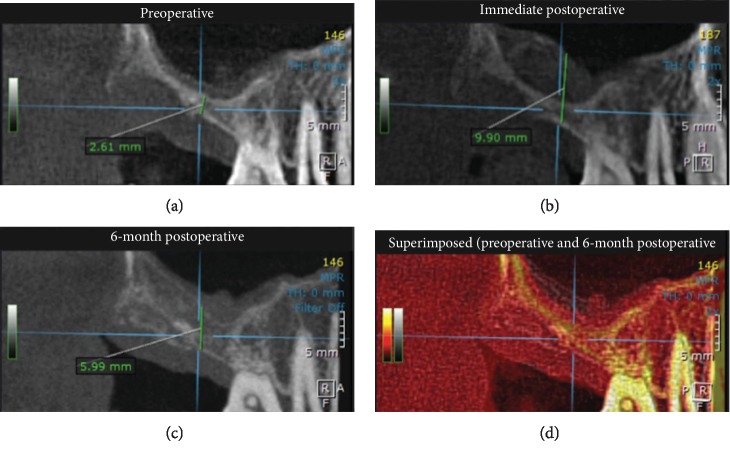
The sagittal view CBCT images show the left posterior edentulous maxillary region of the patient at preoperation (a), immediate postoperation (b), 6-month postoperation (c), and superimposed CBCT image between preoperation and 6-month postoperation of the lateral sinus augmentation (d).
